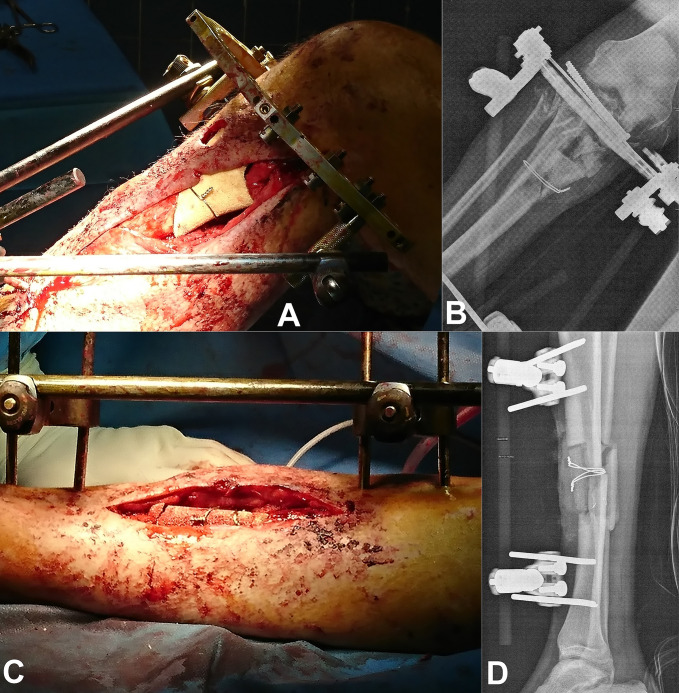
Implantation of the ceramic: A, Intraoperative view of the right tibia, (B) postoperative radiograph of the right tibia, (C) intraoperative view of the left tibia, and (D) postoperative radiograph of the left tibia
